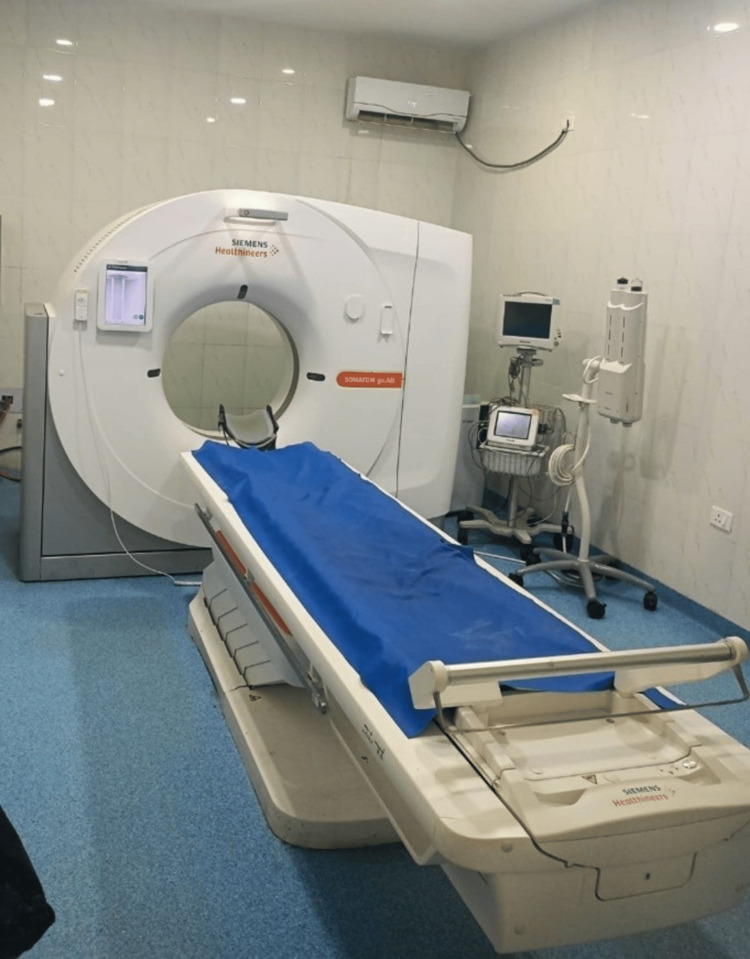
Siemens SOMATOM Go ALL 64-slice multidetector CT scanner used as the reference standard imaging platform. Acquisition parameters: 1.25 mm collimation, 120 kVp, 120 mAs; reconstructed in axial and coronal planes at 3 mm slice thickness. CT = computed tomography
